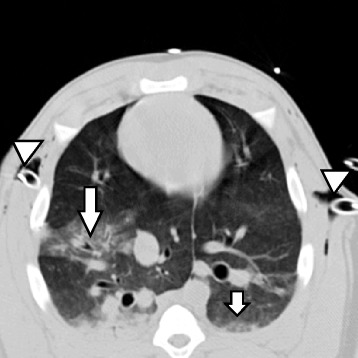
Representative CT image taken during breath-hold at end-inspiratory pressure at the level of the contusion 90 min after the pulmonary contusion. Left and right upside down arrows show the chest tubes used to drain bilateral pneumothoraces. Arrows in the right and left lung show the opacification directly caused by contusion and “contre-coup”, respectively
